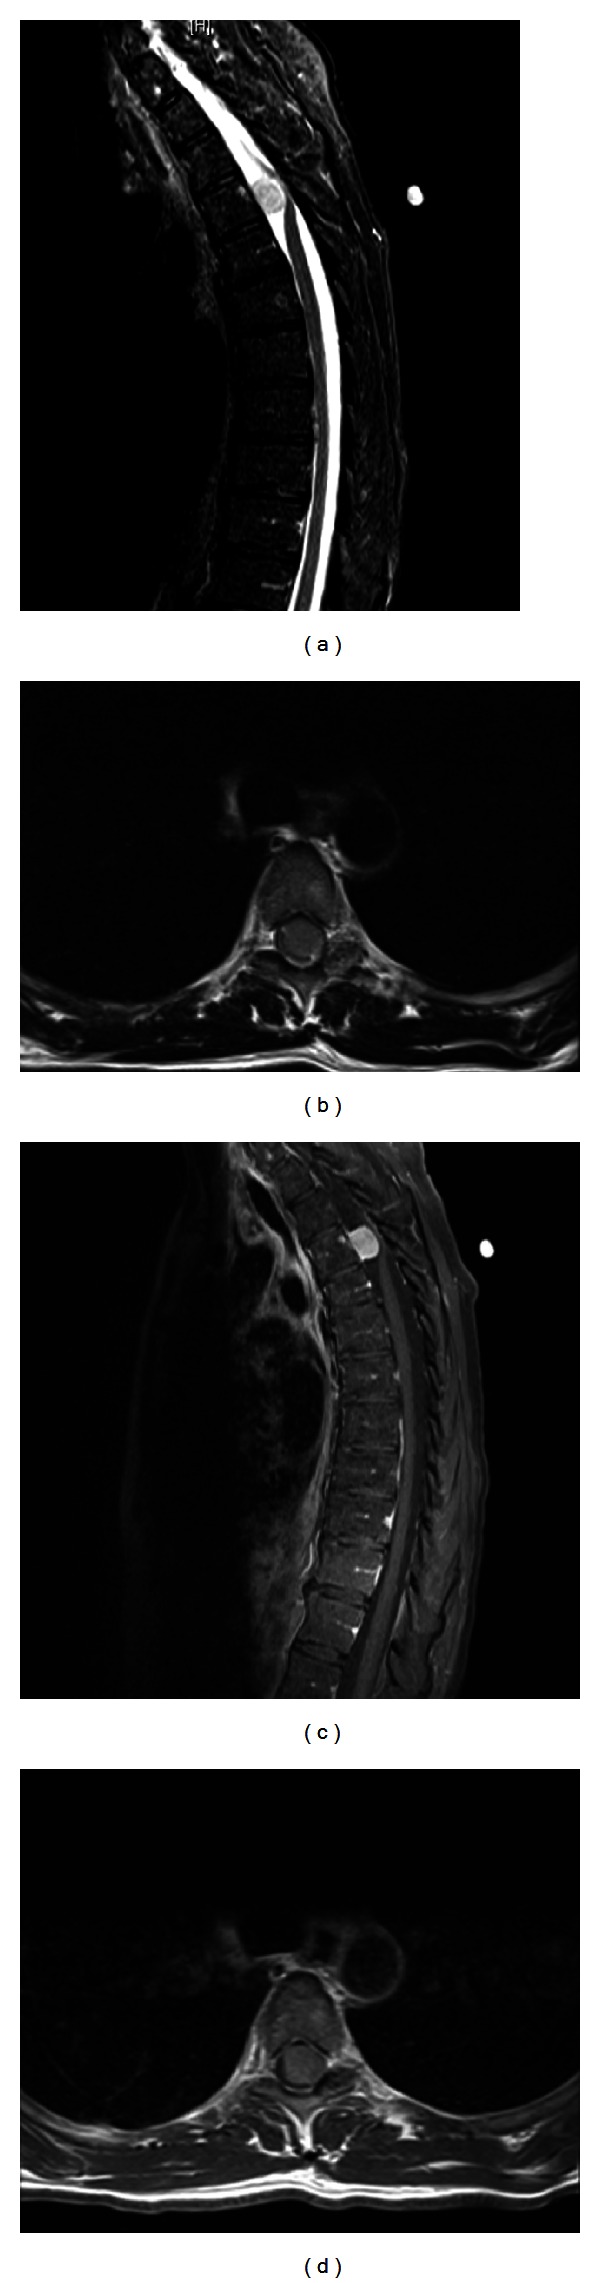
(a) Pre-op sagittal T2 MRI, meningioma at T4 level. (b) Pre-op axial T2 MRI, meningioma at T4 level. (c) Pre-op sagittal T1 MRI with contrast, meningioma at T4 level. (d) Pre-op axial T1 MRI with contrast, meningioma at T4 level.
